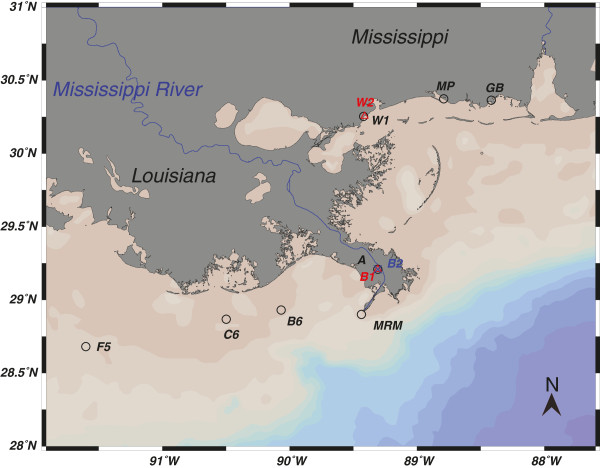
Sampling stations in the northern Gulf of Mexico. Stas. C6, CT, F5 and B6 were from marine shelf; Stas. Waveland H, W1, GB, and MP were from salt marsh; MRM from the Mississippi River mouth; A, B1, B2 were from estuarine freshwater marsh.
